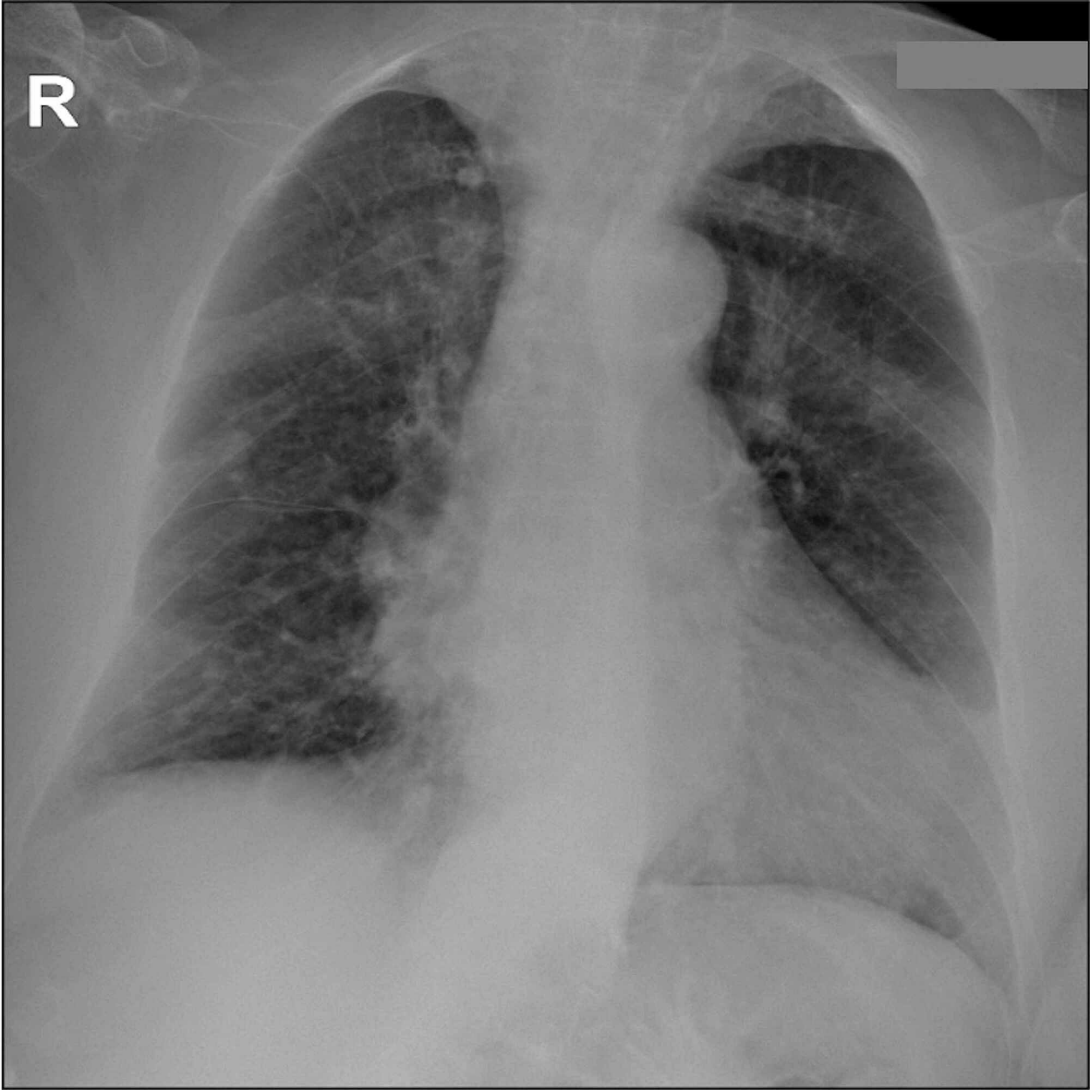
Chest radiograph showing a bilateral reticular pattern.
